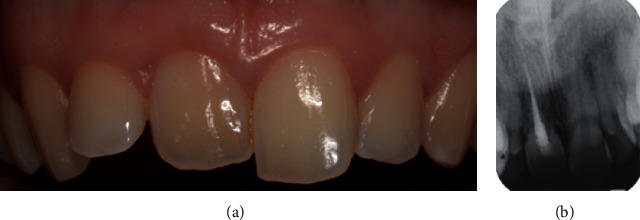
(a) Clinical and (b) radiographic view of the upper incisor at the 8-year follow-up visit: tooth infraposition and root resorption are evident.
